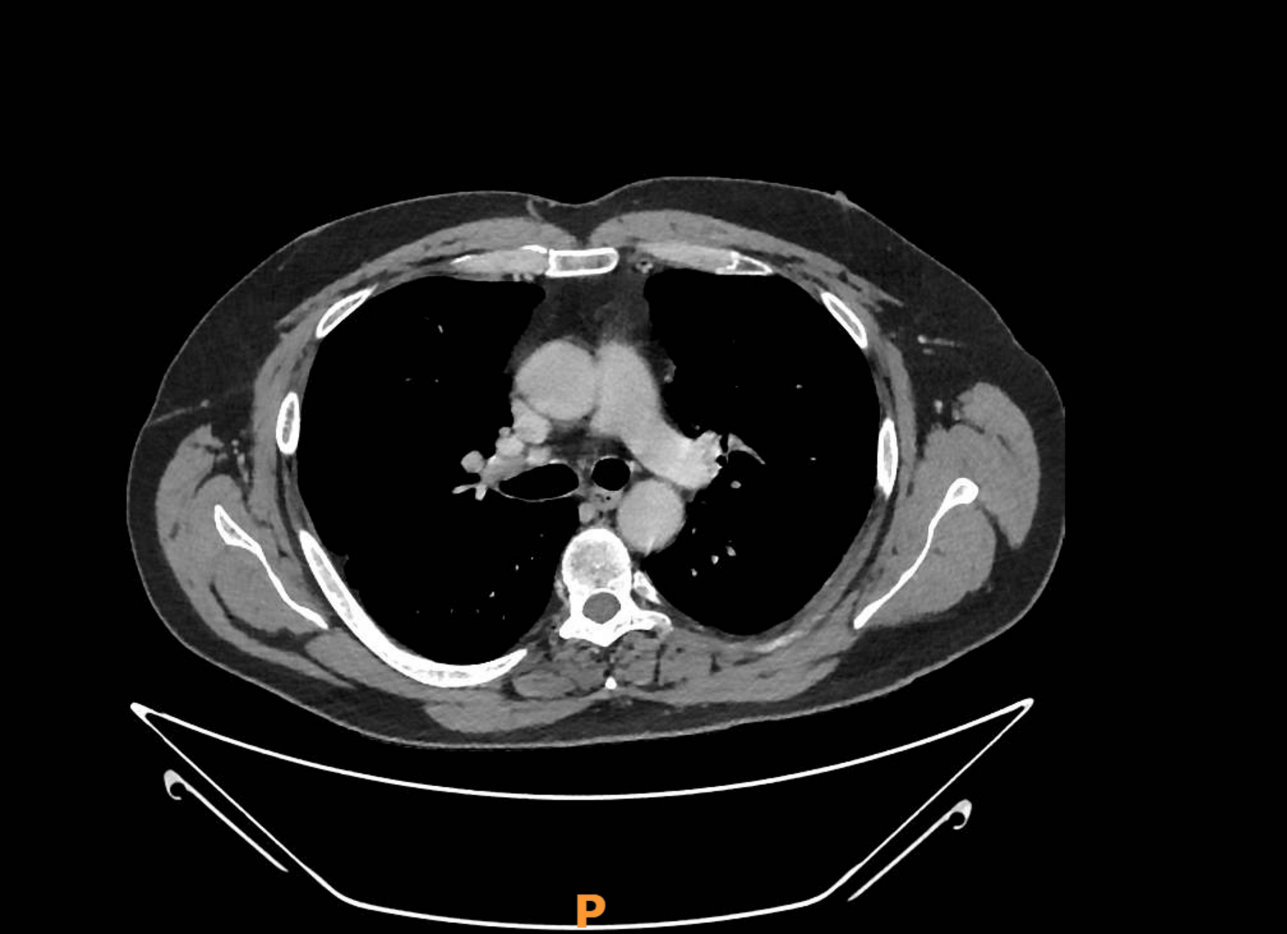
CT scan demonstrating resolution of the previously noted pneumonia in the middle lung zones
